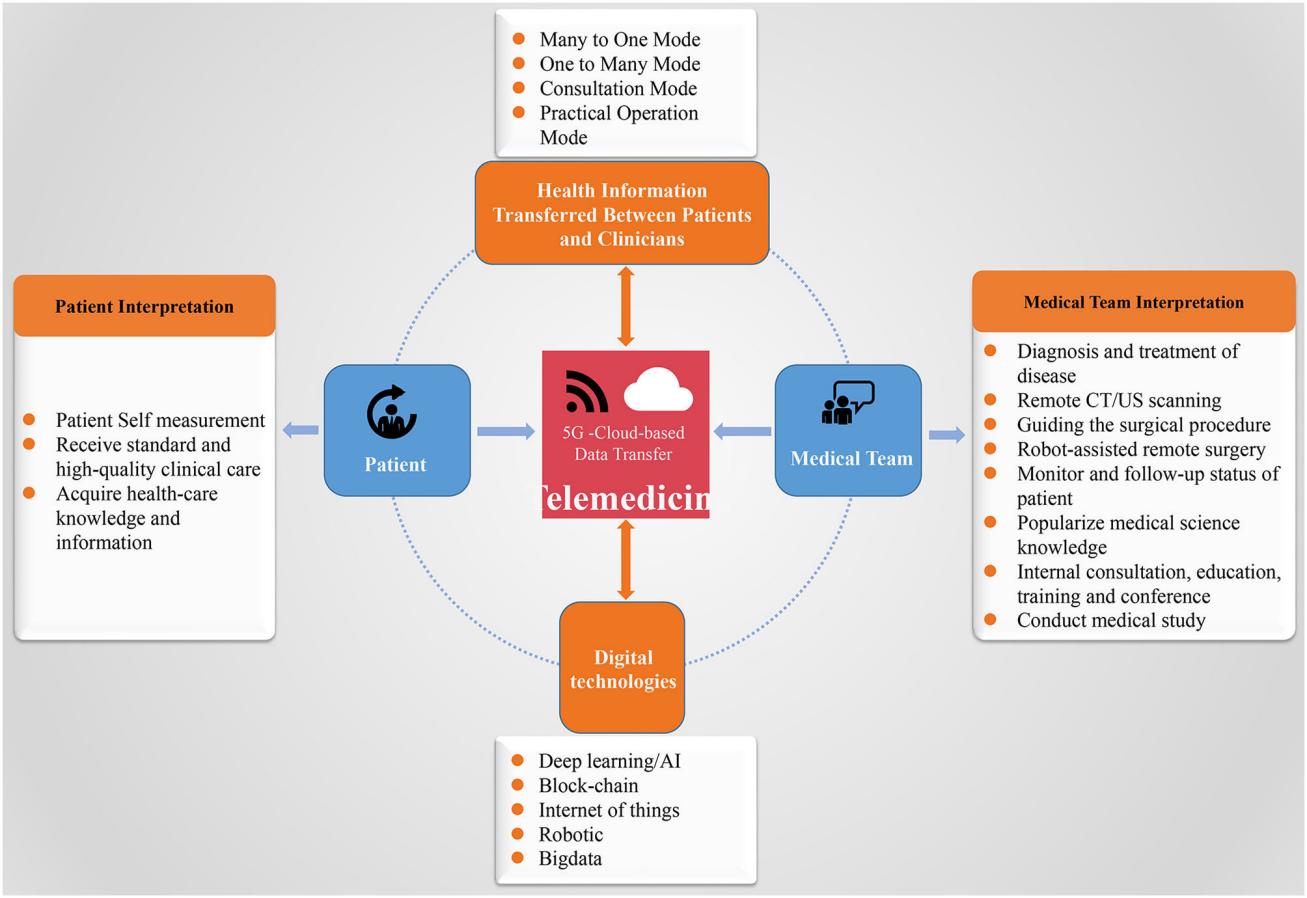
The proposed data flow of telemedicine for clinical care: to maximize clinical care though telemedicine system, a closed loop is quite necessary, which involves healthcare data derived from patients and practitioners; transferred via 5G-Cloud internet; interpreted by the patients, medical practitioners, or with certain automated platforms (e.g., AI, robot, and big data analysis); and returned back to the patients and medical staff for better clinical decisions. And in turn, the large-scale shared cloud-based data would provide a great opportunity for AI development. 5G, fifth generation; AI, artificial intelligence; CT, computed tomography; US, ultrasound.
